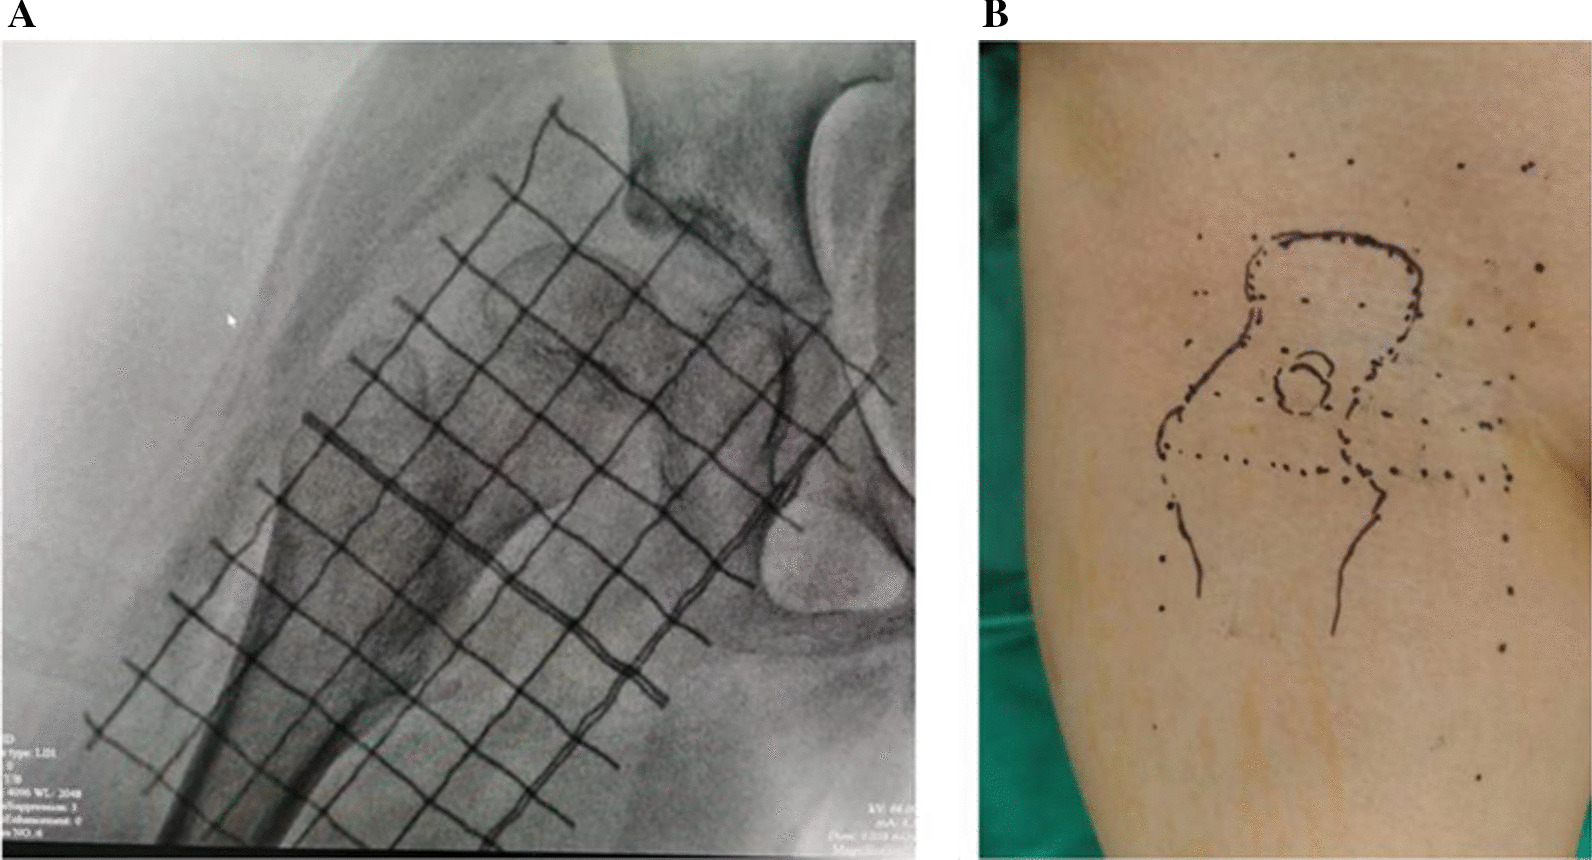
The grid localization method was used to locate the lesions: Grid positioning under X-ray (A) and body surface marking (B）
